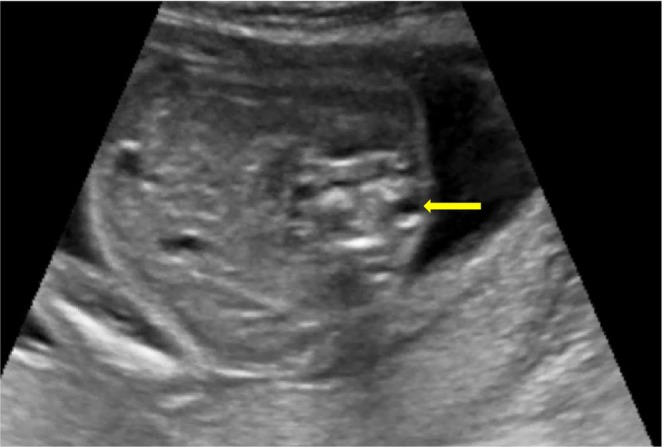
Ultrasound image of fetal spine in axial view showing the absence of a right segment of a vertebra (yellow arrow).
